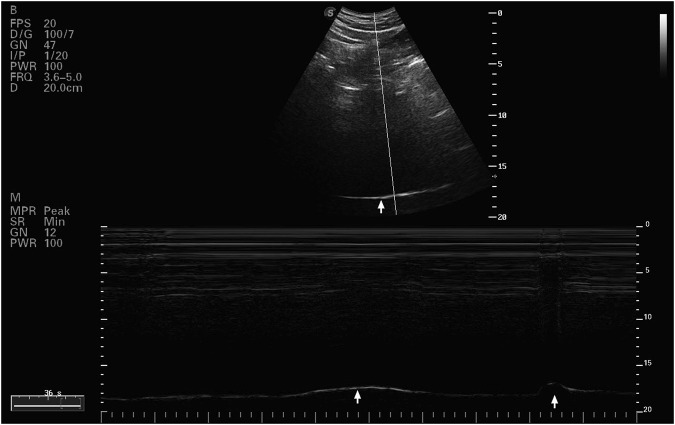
Ultrasound B and M mode image of rigth diaphragmatic dome.
